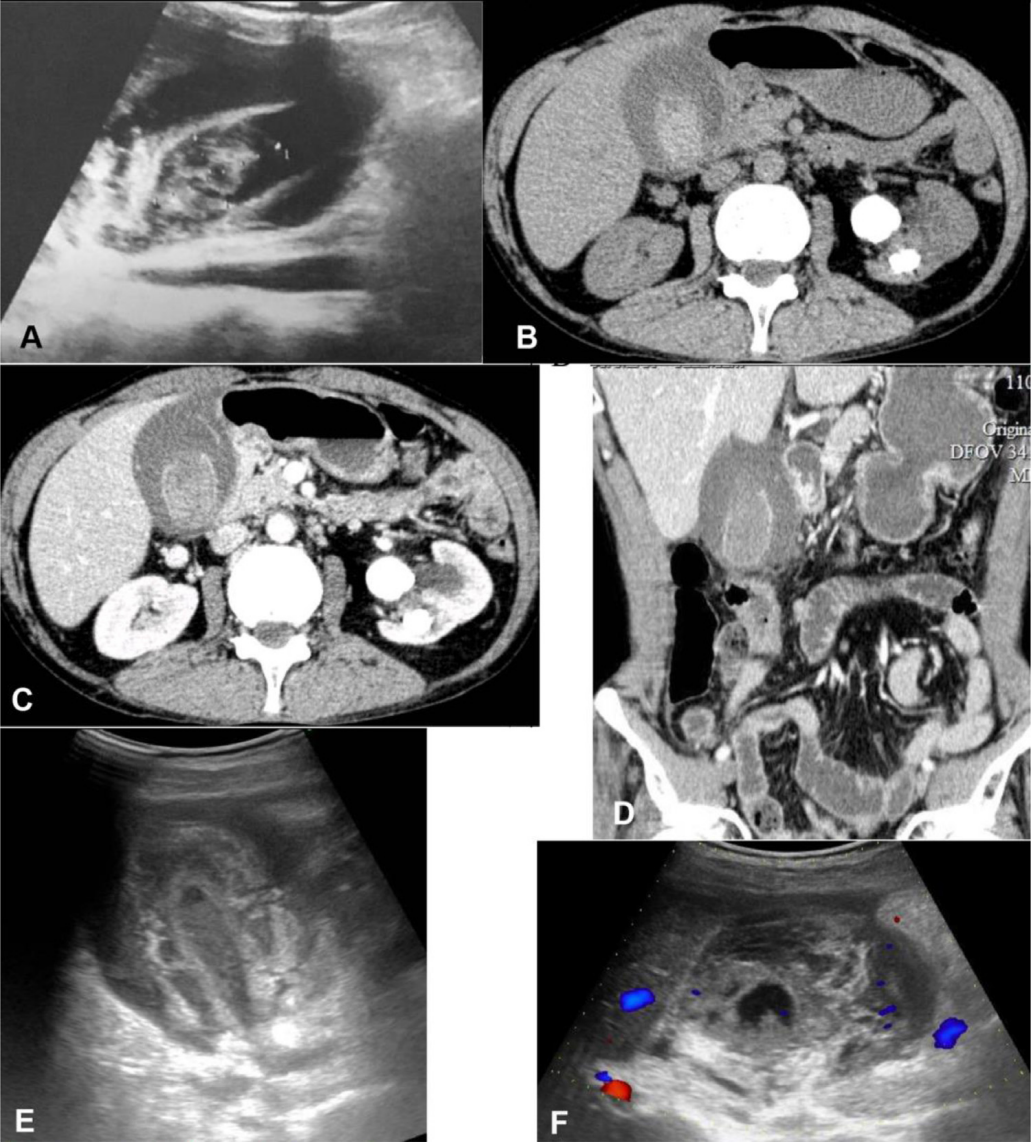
A few hours after hopitalisation, galblladder ultrasound (US) (A) showed a thickned wall, heterogenous material of intraluminal blood clot and pericholecystic fluid; Computed tomography (CT) confirmed a high density of intraluminal bloody clots on non-contrast CT (B) and intramual disection with discontinuity of the enhanced mucosal wall located on the liver bed (C and D). On day 4 post admission, US showed a diffuse gallbladder wall thickening (E) and avascularity on color Doppler (F) surrounded by pericholecystic fluid thickness of 23 mm and an echogenic blood level in the lumen.
